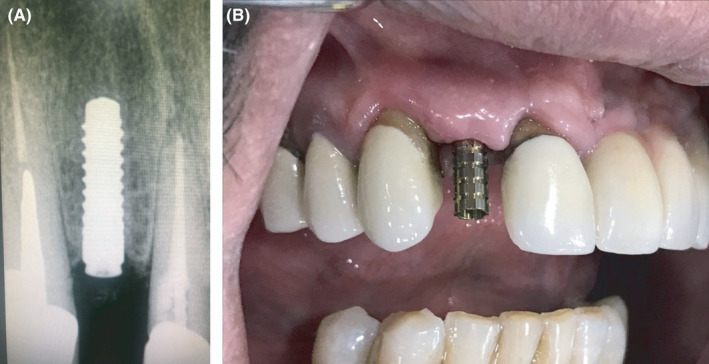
(A) Intraoral periapical radiograph and 1 (B) seating the temporary abutment
